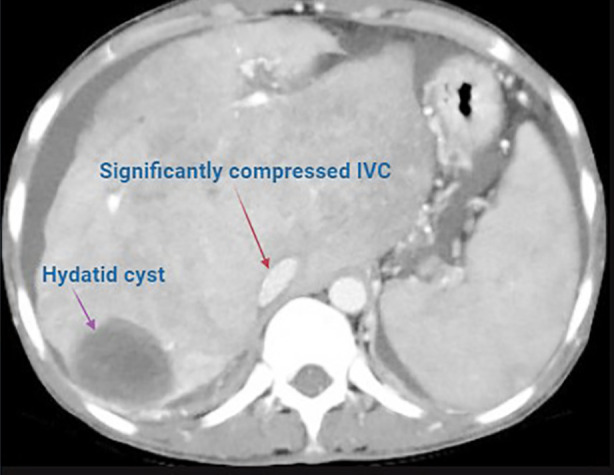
Preoperative Triphasic CT Abdomen Showing Features of Chronic Budd-Chiari Syndrome. Triphasic CT abdomen demonstrating heterogeneous hepatic parenchymal enhancement with caudate lobe hypertrophy, thrombosed right hepatic vein, and non-visualized middle and left hepatic veins. Other findings are moderate-to-severe ascites, segment VII hydatid cyst, tiny hypervascular regenerative nodules in the right lobe, and an eccentric bland thrombus spanning the right portal vein to the main portal vein up to the junction of the portomesenteric. A small thrombus is also seen in the right renal vein near its drainage into the inferior vena cava.
